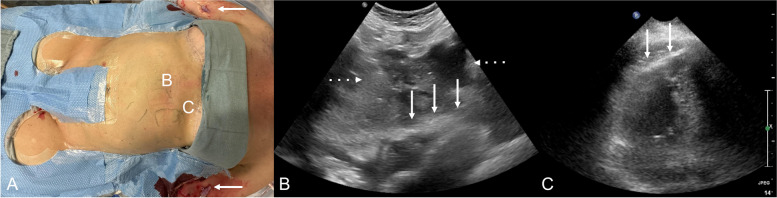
(A) Example of patient preparation for TCVO recanalization cases; white arrow indicating bilateral upper extremity access sites. “B” indicates subxiphoid view location and “C” indicates parasternal view location corresponding with images B and C location. (B) Subxiphoid echocardiogram view with white arrows indicating pericardial space with no effusion. Liver with multifocal metastasis (dashed arrows). (C) Parasternal echocardiogram view with arrows indicating pericardium. If pericardial drain was indicated, a parasternal approach would be preferred to avoid hepatic transgression
